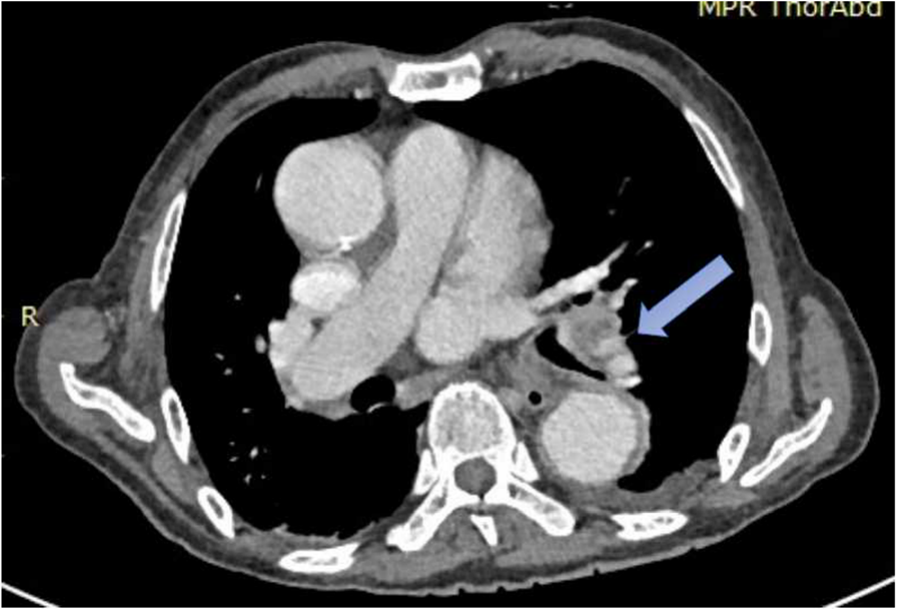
Axial view of contrasted computed tomography scan of the thorax shows an enlarged left hilar lymph node (*blue arrow*)
